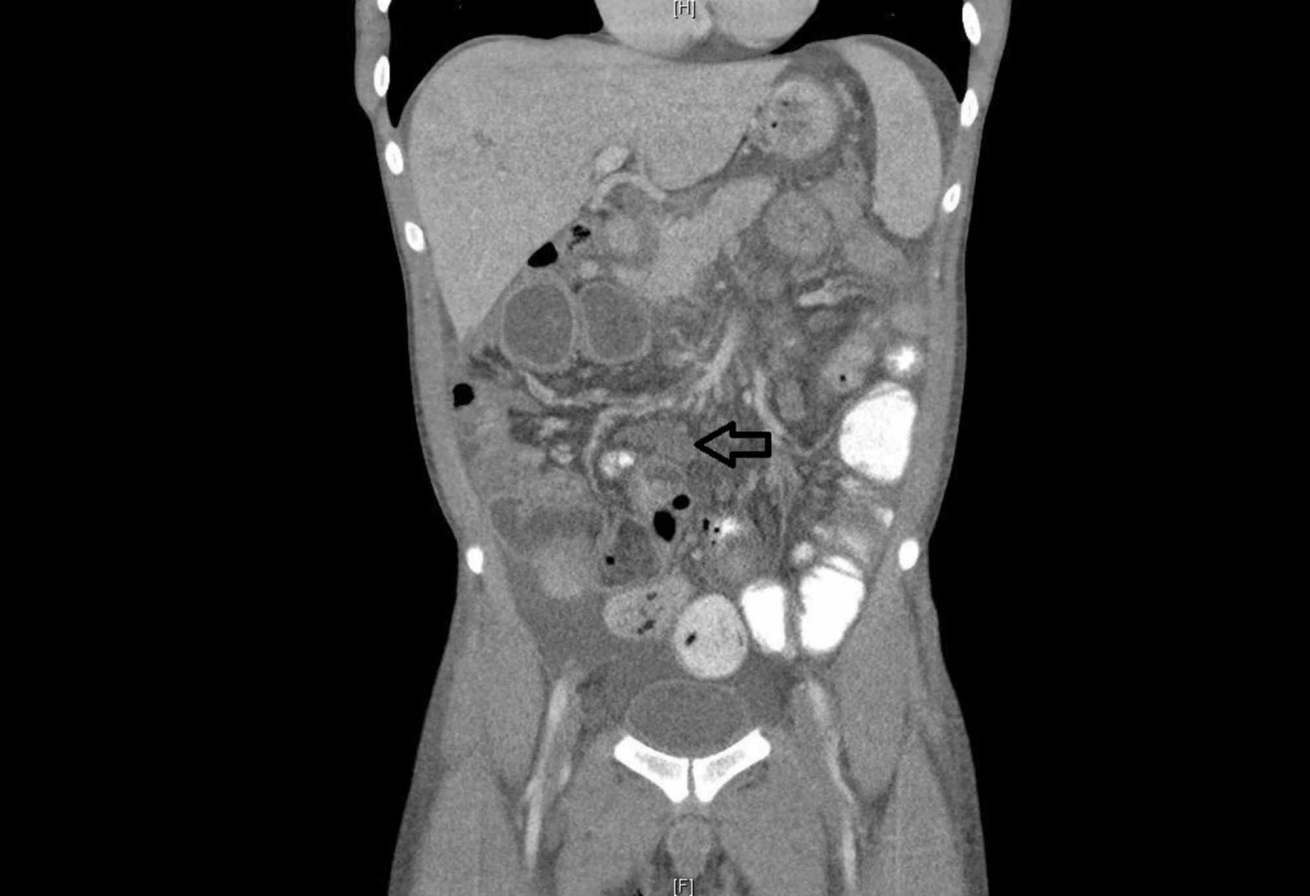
Sagittal section of CT abdomen/pelvis showing enlarged somewhat necrotic mesenteric lymph node (arrow).
